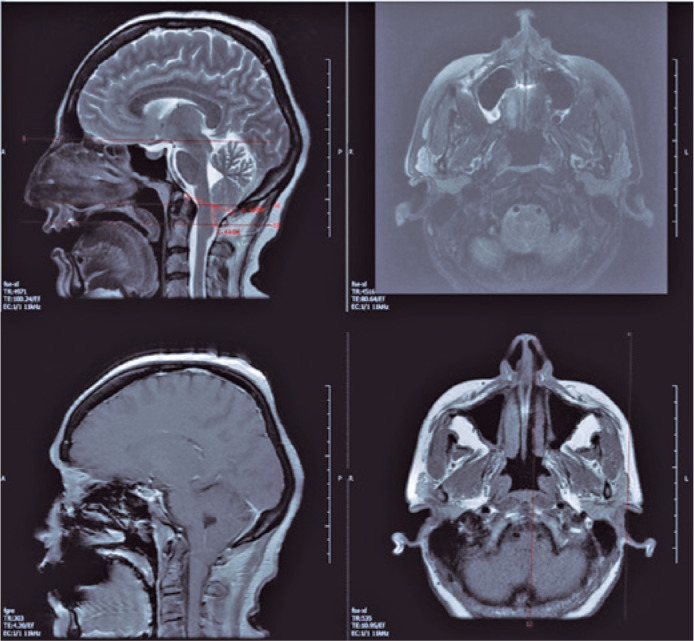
Brain magnetic resonance imaging with contrast; images of sagittal and axial
